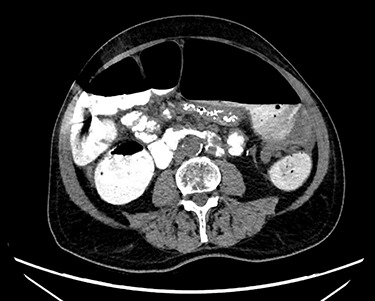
CT scan demonstrating a dilated colonic loop with air-fluid levels and swirling of the mesentery, indicative of a large bowel obstruction secondary to a volvulus.
